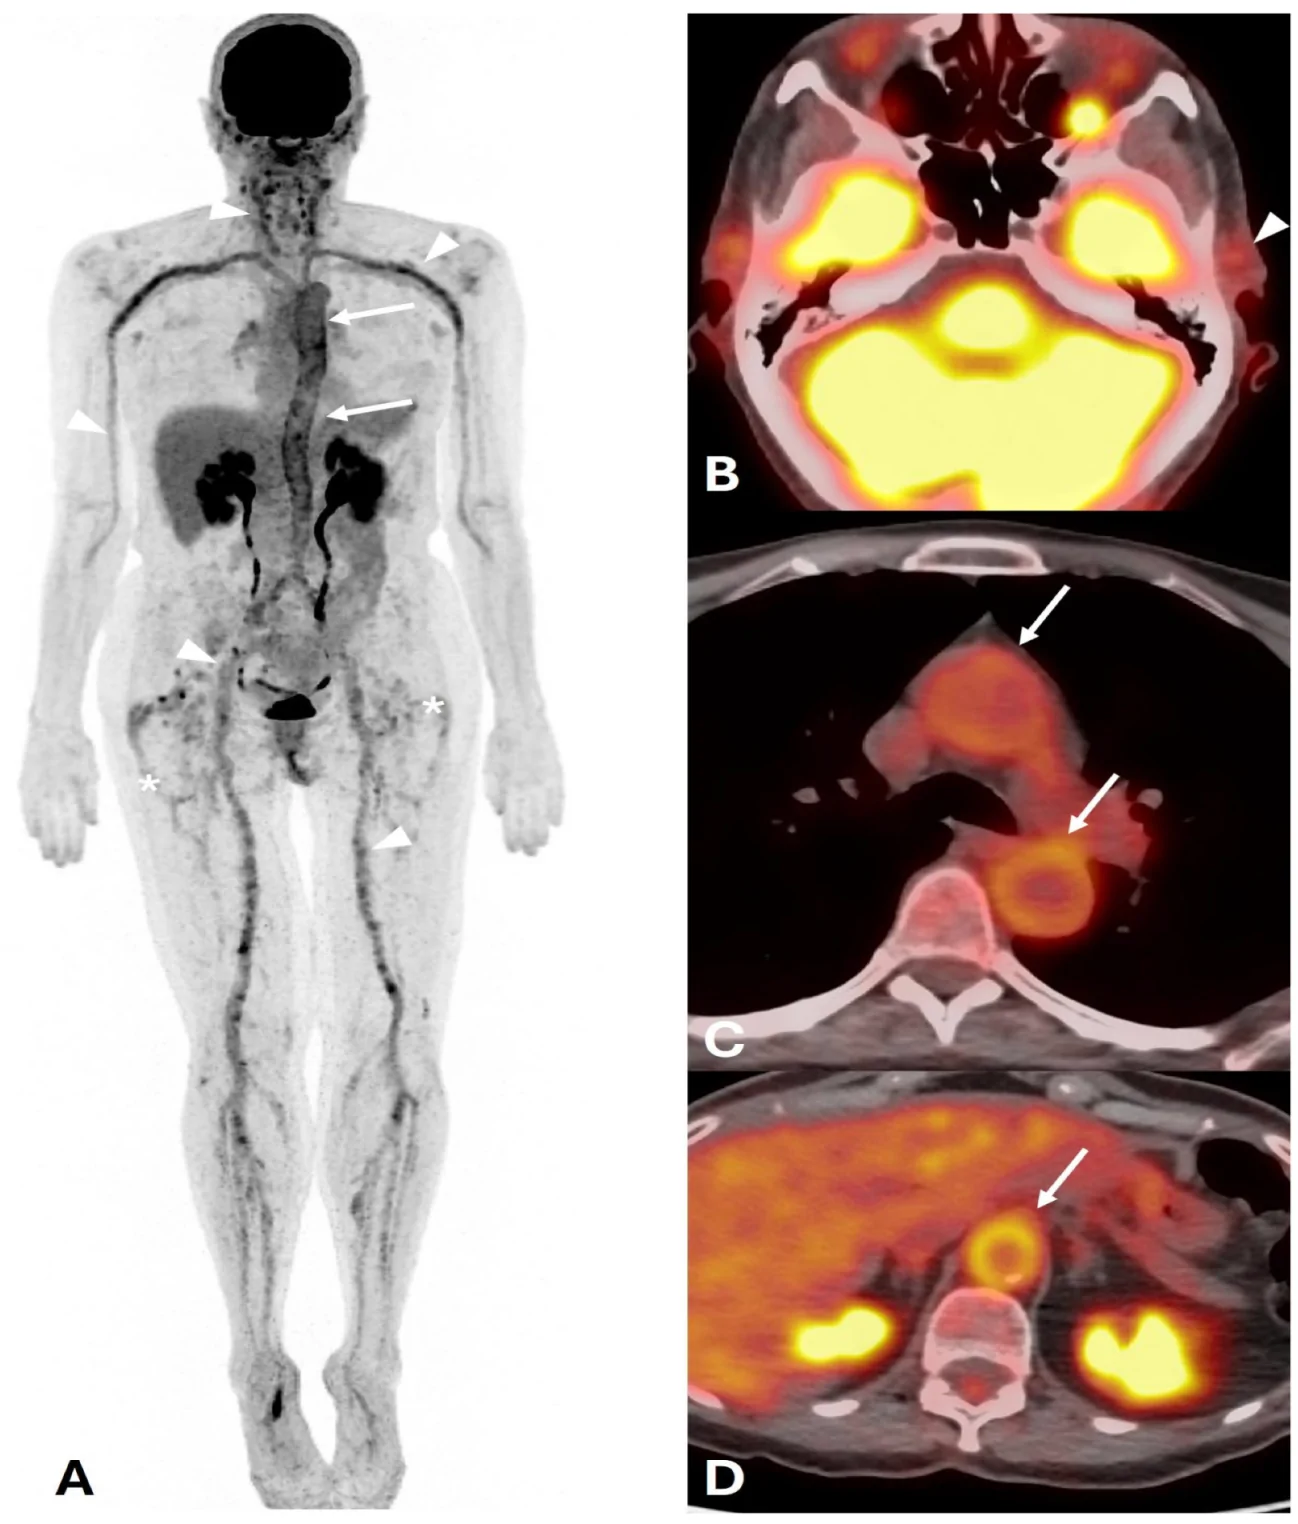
Patient with known giant cell arteritis. Images of whole-body F-18 FDG PET/CT, PET MIP (A) and axial fused images at multiple levels (B–D) showing extensive abnormal tracer activity involving the aorta (arrows), as well as head and neck vessels, upper and lower extremity arteries and superficial temporal arteries (arrowheads) indicating active vasculitis. In addition, multifocal areas of abnormal tracer accumulation in lower extremities musculature most consistent with active myositis (*).
